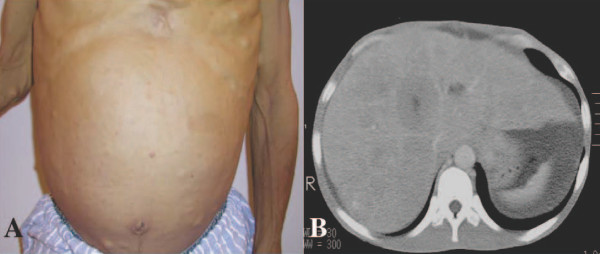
Examinations on admission. (A) Multiple discrete cutaneous neurofibromas and café au lait spots located on his abdominal wall. The abdominal wall was swollen with ascites. (B) An abdominal CT study showed multiple liver lesions and ascites.
